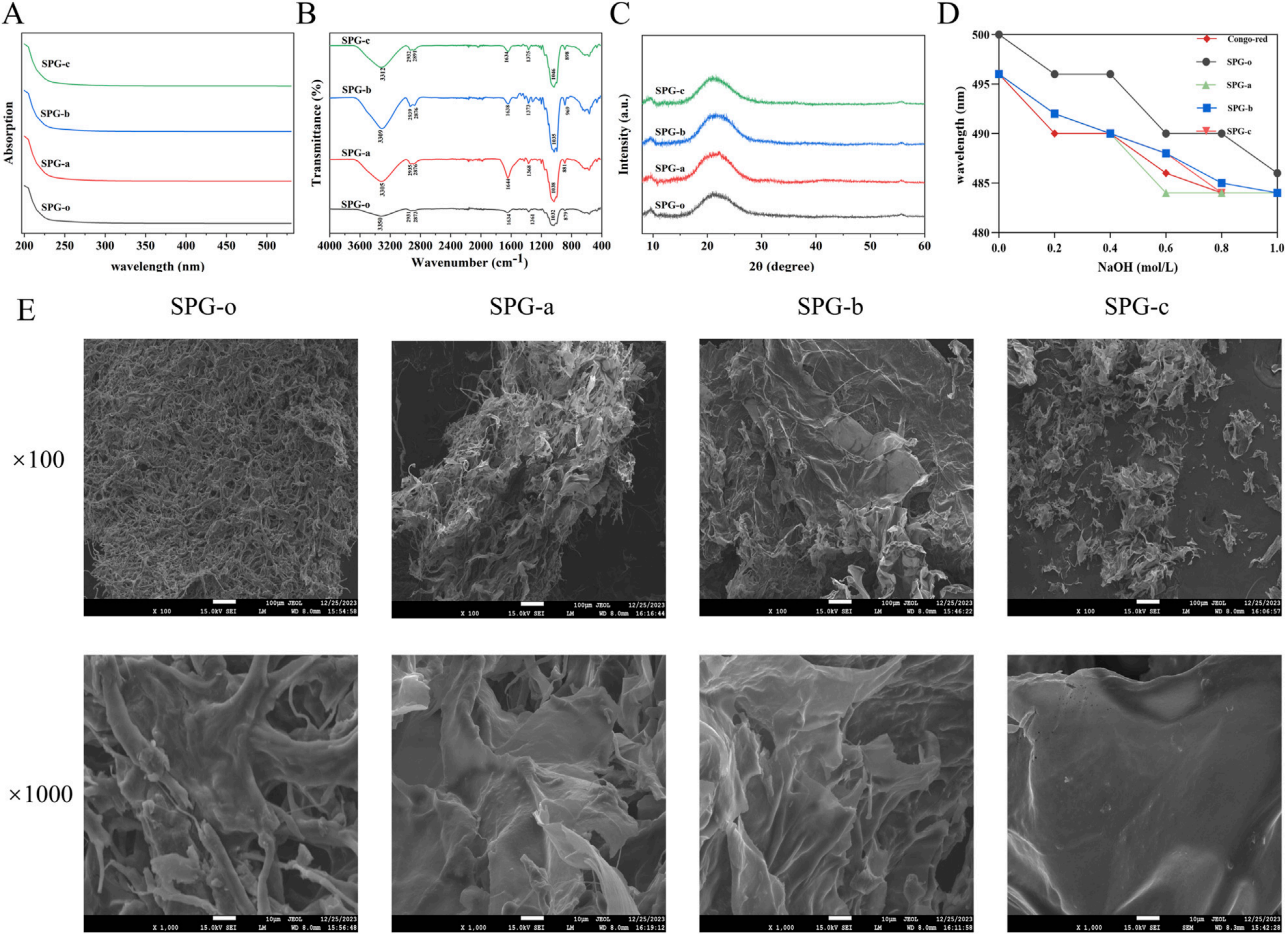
Structural characterization of SPG. (A) UV-Vis spectra. The analysis showed that all SPG samples were absent of nucleic acid and protein impurities. (B) FT-IR spectra. Revealing characteristic polysaccharide absorption peaks and confirming the preservation of pyranose-type polysaccharides and β-glycosidic bonds after degradation. (C) XRD spectra. Indicating SPG’s predominantly amorphous structure, which remains unchanged after ultrasonic-assisted H_2_O_2_ degradation. (D) Congo red interaction assay. The triple helical structure of the degraded SPG samples was disrupted. (E) SEM images. Showing changes in surface morphology. SPG-o: Undegraded SPG, Mw = 4,409,608 Da. SPG-a: Degraded SPG, Mw = 257,500 Da. SPG-b: Degraded SPG, Mw = 429,300 Da. SPG-c: Degraded SPG, Mw = 364,800 Da.
